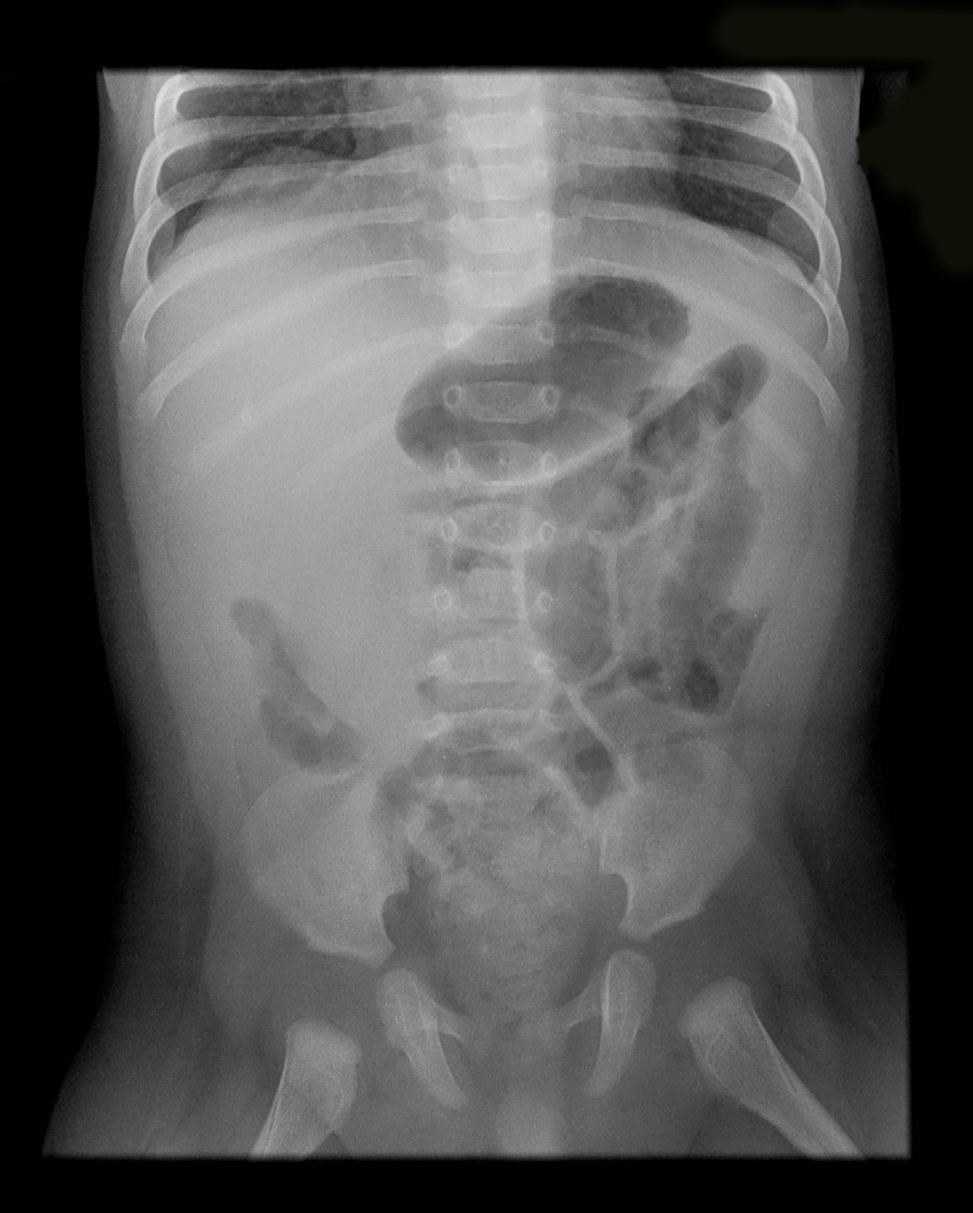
Abdominal X-ray showing small bowel obstruction.
